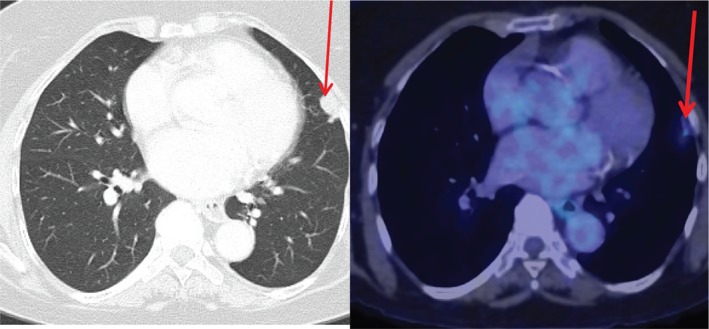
High‐resolution computed tomography chest images (left) and computed tomography‐positron emission tomography images (right) showing nodule, highlighted with arrow.
